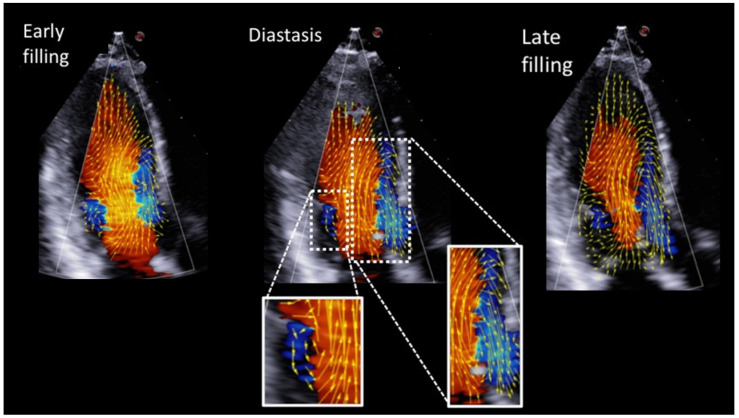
Apical long-axis views with flow velocity vector maps showing mitral inflow during early filling, diastasis and late filling with a pair of counterrotating vortices, one distal to the anterior mitral valve leaflet (larger vortex) and one distal to the posterior leaflet (smaller vortex). Velocity vectors are overimposed on the standard color Doppler flow representation. The anterior and posterior vortices are magnified for better appreciation.
